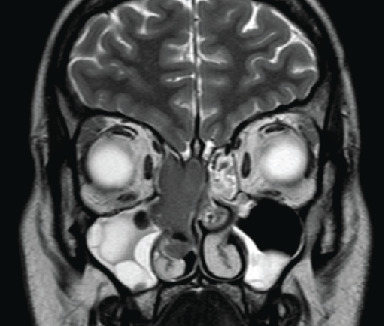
Contrast-enhanced MRI sinuses (coronal section, T2-weighted image). Demonstrates the demarcation between the mass and polypoid maxillary sinus, not appreciated on CT.
